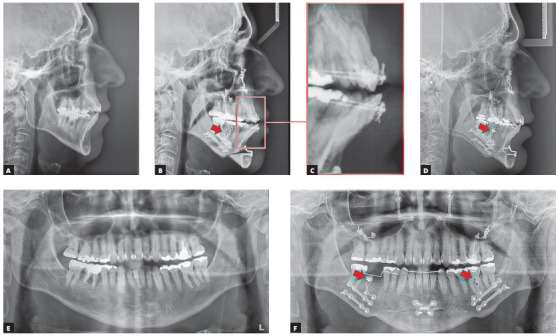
Cephalometric radiographs: initial (A) and immediately after surgery (B). Note that the mandibular advancement was greater than the maxillary advancement, leading to an anterior crossbite (C). This relationship was corrected by means of skeletal anchorage miniplates, which provided the necessary anchorage for lower arch retraction (arrows in B, D and F). The right lower first molar was removed before surgery (E). Panoramic radiographs: initial (E) and immediately after surgery (F).
